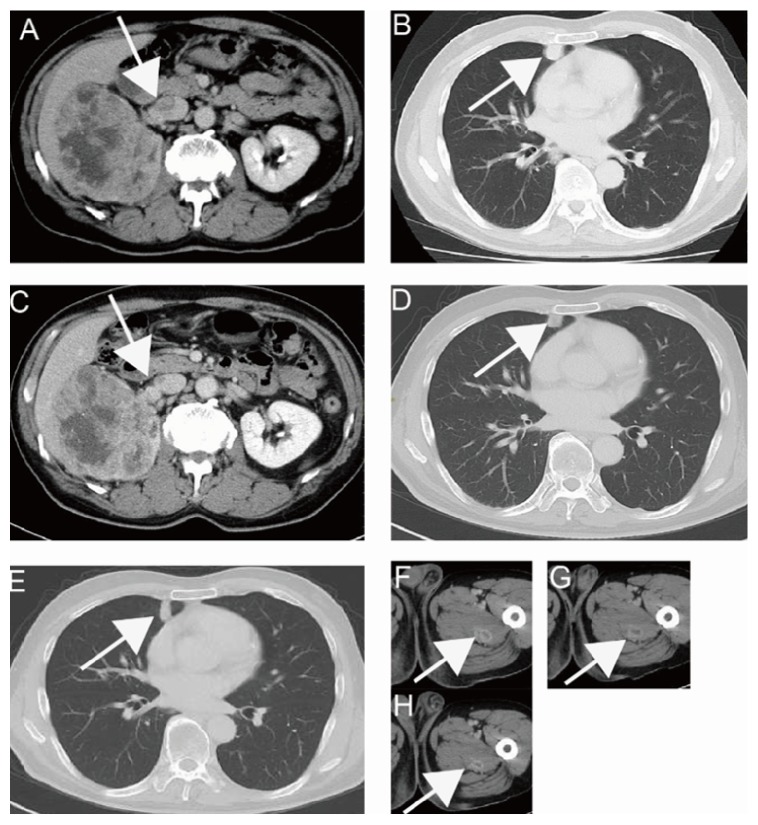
Computed tomography (CT) finding of primary site (A, C), lung metastasis (B, D, E), and intramuscular metastasis (F-H)*. Hypervascular renal tumor with liver invasion, IVC extension (arrowhead, left), and lung metastasis (arrowhead, right) were observed. After a month of presurgical treatment, tumor thrombus and lung metastasis had decreased (A-B, F: before treatment, C-D, G: after treatment). CT appearance of lung metastasis and that of intramuscular metastasis at 18 months after surgery are shown (E, H).
